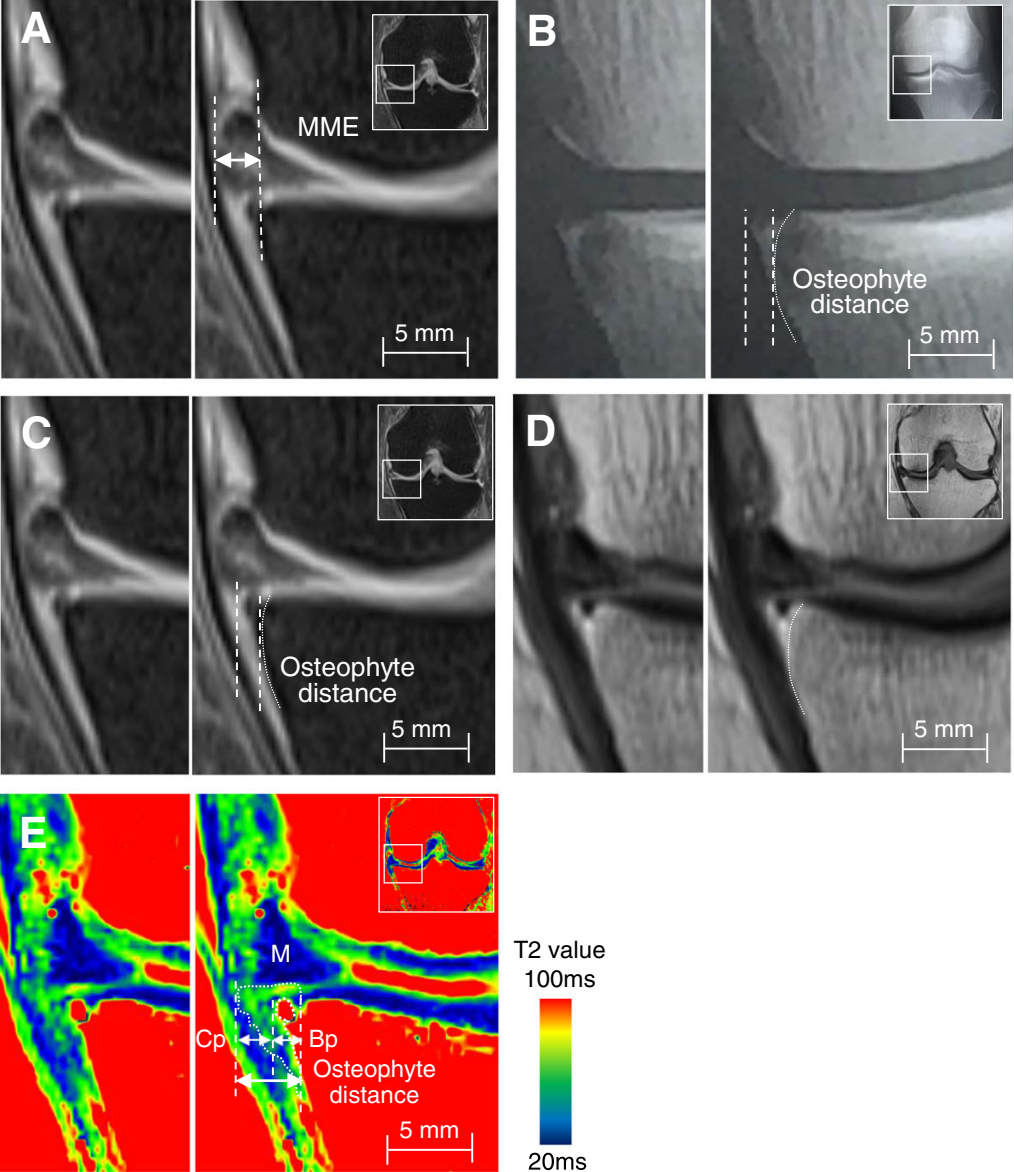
Representative data derived from the measurement of medial meniscal extrusion (MME) and medial tibial osteophyte distance by radiography, proton density-weighted magnetic resonance imaging (MRI), and T2 mapping MRI in the early-stage knee osteoarthritis joint. a Coronal fast spin echo proton density-weighted MRI with fat suppression. MME distance was measured as the distance from the outermost edge of the medial meniscus to a line connecting the femoral and tibial cortices. b Osteophyte distance measurement using radiography. c Osteophyte distance measurement using proton density-weighted MRI with fat suppression. d Definition of a boundary between the tibial cortex and osteophyte (high signal zone) using proton density-weighted imaging without fat suppression. e Osteophyte distance measurement using T2 mapping MRI. *Dashed lines* indicate cartilage and bone parts of the osteophyte. The line between bone part of osteophyte and tibial cortex was drawn by tracing the image obtained by proton density-weighted MRI without fat suppression. *Bp* Bone part of osteophyte, *Cp* Cartilage part of osteophyte, *M* Meniscus
